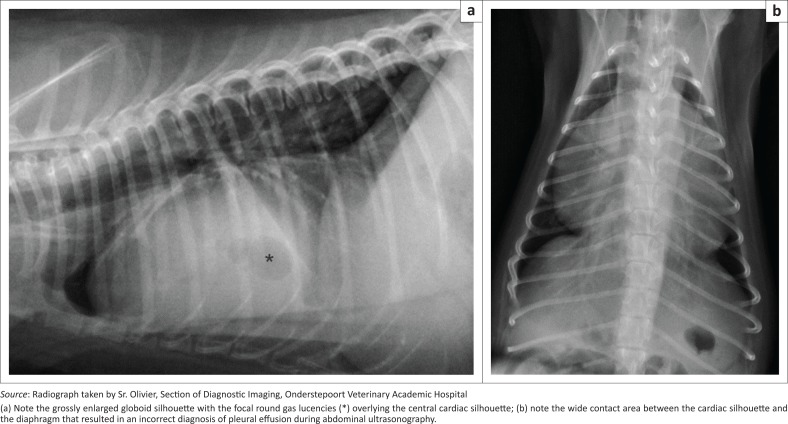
Right lateral (a) and DV (b) thoracic radiographs.
